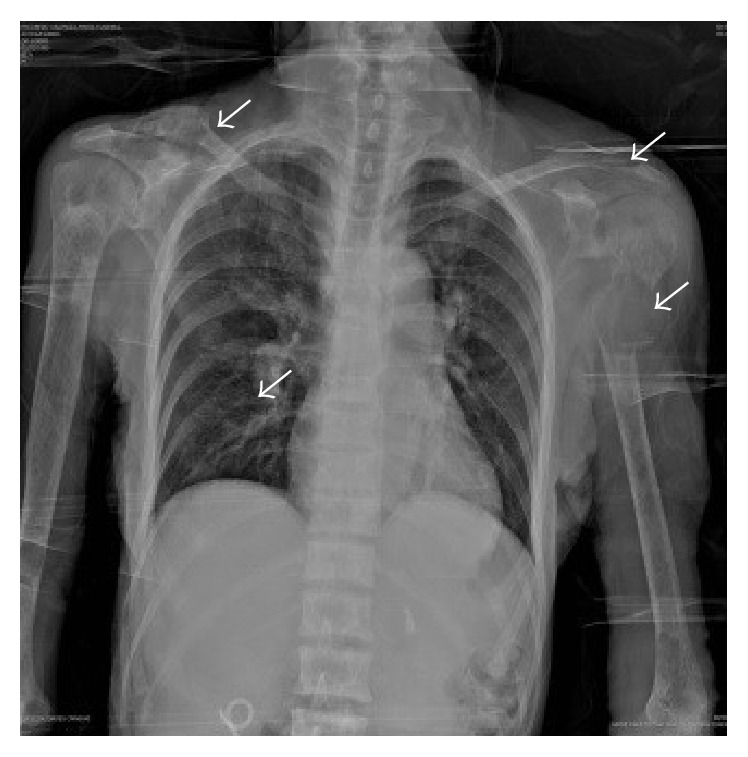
Chest X-ray with severe widespread cortical bone loss, cysts, and brown tumors (arrows) in ribs, distal third of the clavicle, and humerus for severe cystic fibrous osteitis due to prolonged PHPT.
